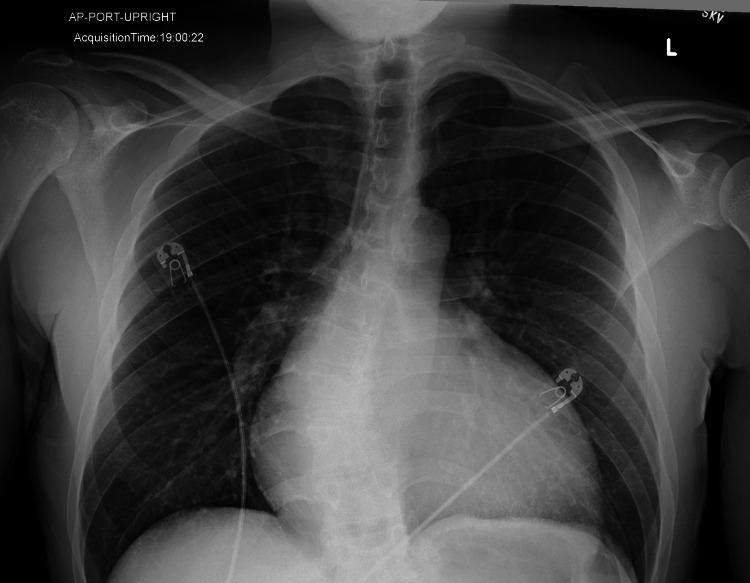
Chest X-ray upon admission Mild interstitial prominence in the lungs related to mild pulmonary vascular congestion. An enlarged cardiac silhouette is noted, consistent with cardiomegaly.
